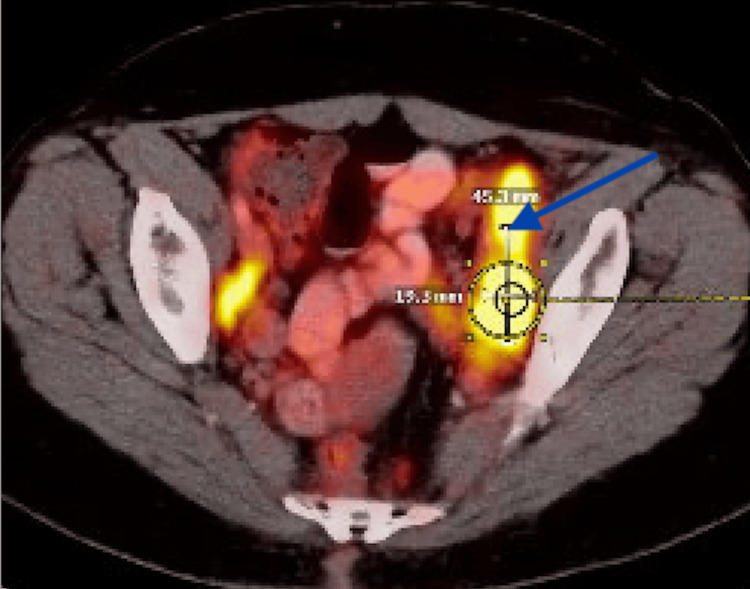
PET-CT scan Blue arrow shows intensely fludeoxyglucose-18 (FDG)-avid pelvic lymphadenopathy.
